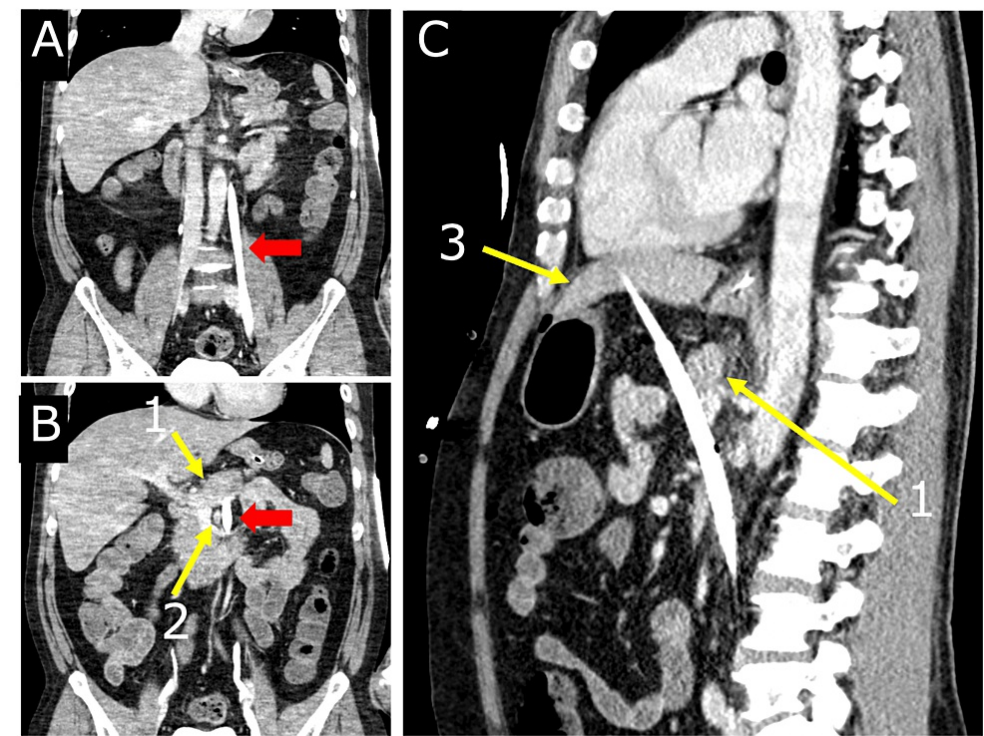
Contrast-enhanced CT (A) The venous cannula (red arrow) had strayed into the left ascending lumbar vein and perforated the retroperitoneal space. (B) The venous cannula (red arrow) had passed between the pancreas and the horizontal portion of the duodenum. (C) The tip of the venous cannula appeared to be impaled in the hepatic left lateral lobe. 1: Pancreas, 2: Superior mesenteric artery, 3: Liver
